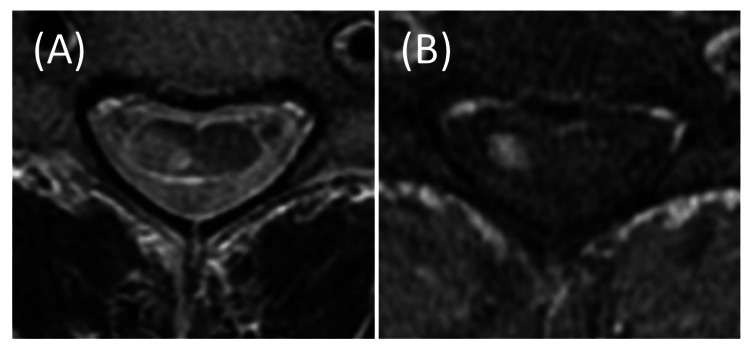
Cervical cord contrast-enhanced MRI in Patient 1 Axial T2-weighted (A) and T1 contrast-enhanced (B) images on admission. On T2-weighted image (A), a high signal intensity area was observed on the right side of the cervical spinal cord, with corresponding gadolinium enhancement in the same region (B). These findings are indicative of acute myelitis.
